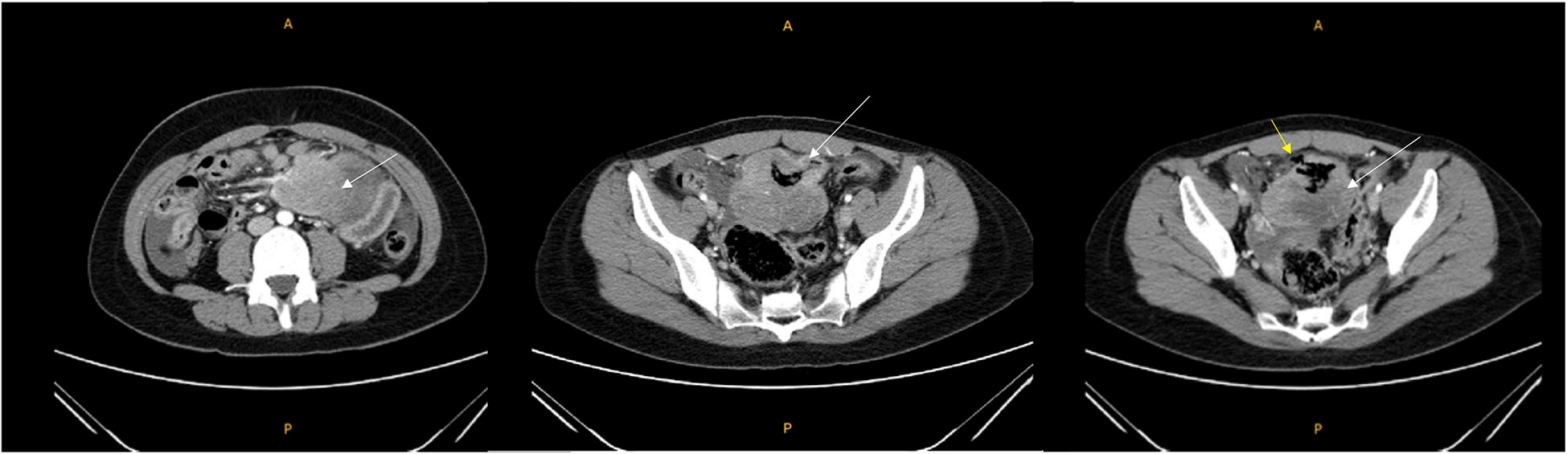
Initial CT scan was notable for multiple huge masses (White arrow: mass, Yellow arrow: perforation site)
